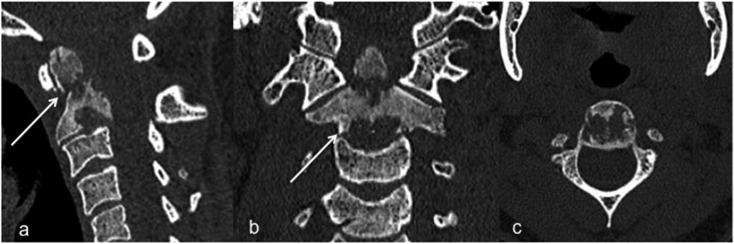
(a) Sagittal, (b) coronal and (c) axial CT-scan reformations of the upper cervical spine in a 30-year-old woman, showing the mixed appearance of the C2 vertebral body with a ground-glass appearance and osteolytic areas in the base of the odontoid process with a pathologic fracture (thin arrow) and in the inferior part of the vertebral body with partial destruction of the inferior endplate (thick arrow).
